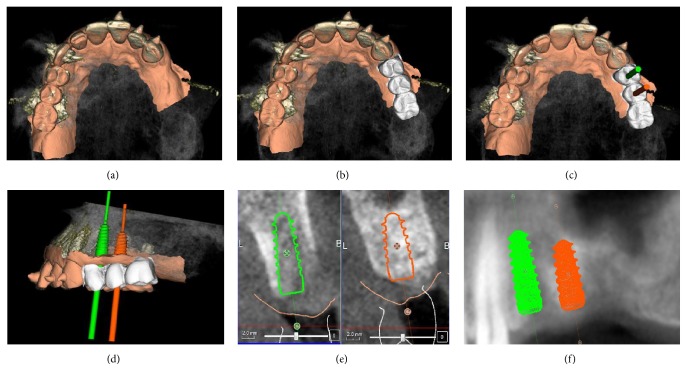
Surgical and prosthetic 3D planning: (a) overlapping of intraoral scan and CBCT; (b) overlapping of intraoral scan and CBCT with modelled provisional restorations; (c) overlapping of intraoral scan and CBCT with modelled provisional restoration and implant planning (occlusal view); (d) overlapping of intraoral scan and CBCT with modelled provisional restoration and implant planning (side view); (e) implant planning (cross sections); (f) implant planning (panorex).
